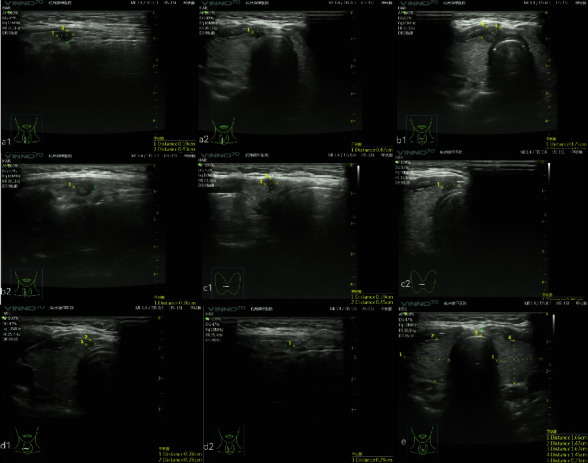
Ultrasound images before and after RFA: (a1-a2) the tumor had a volume of 55.83 mm^3^ before RFA; (b1-b2) the ablated tissue had a volume of 164.27 mm^3^ at 1 month after RFA; (c1-c2) the volume of the ablated tissue decreased to 36.78 mm^3^ at 3 months after RFA; (d1-d2) the volume of the ablated tissue decreased to 11.91 mm^3^ at 6 months after RFA; and (e) the tumor was completely absorbed at 12 months after RFA.
